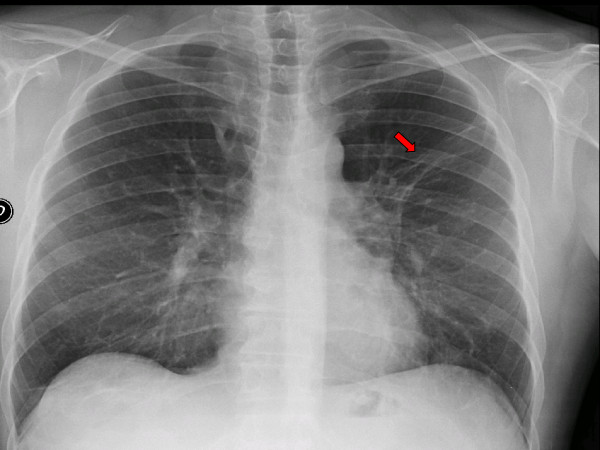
Chest X-ray. Thickening areas and parenchymal distortion can be seen on the left upper lobe. Diffuse thickening can be seen on medial and lower lobes (arrow).
